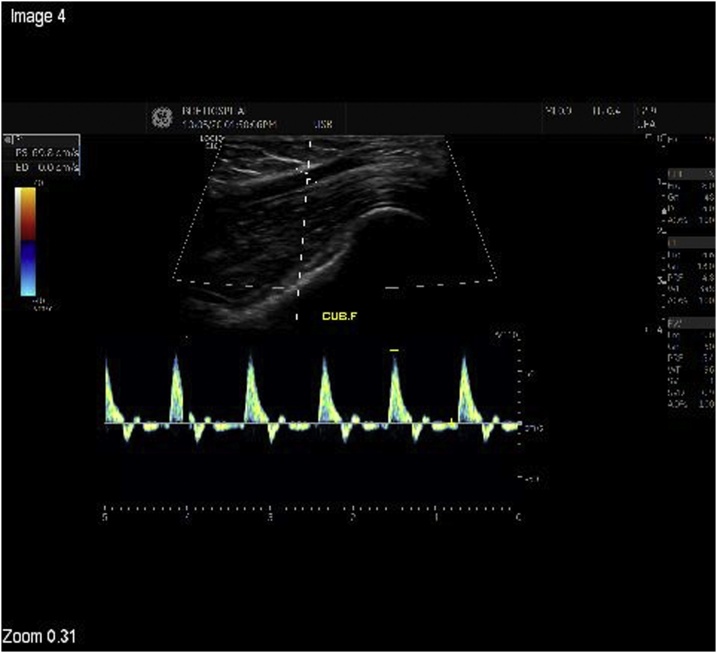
Follow up graft duplex ultrasound showing satisfactory flow without evidence of stenosis at the proximal or distal anastomoses.
